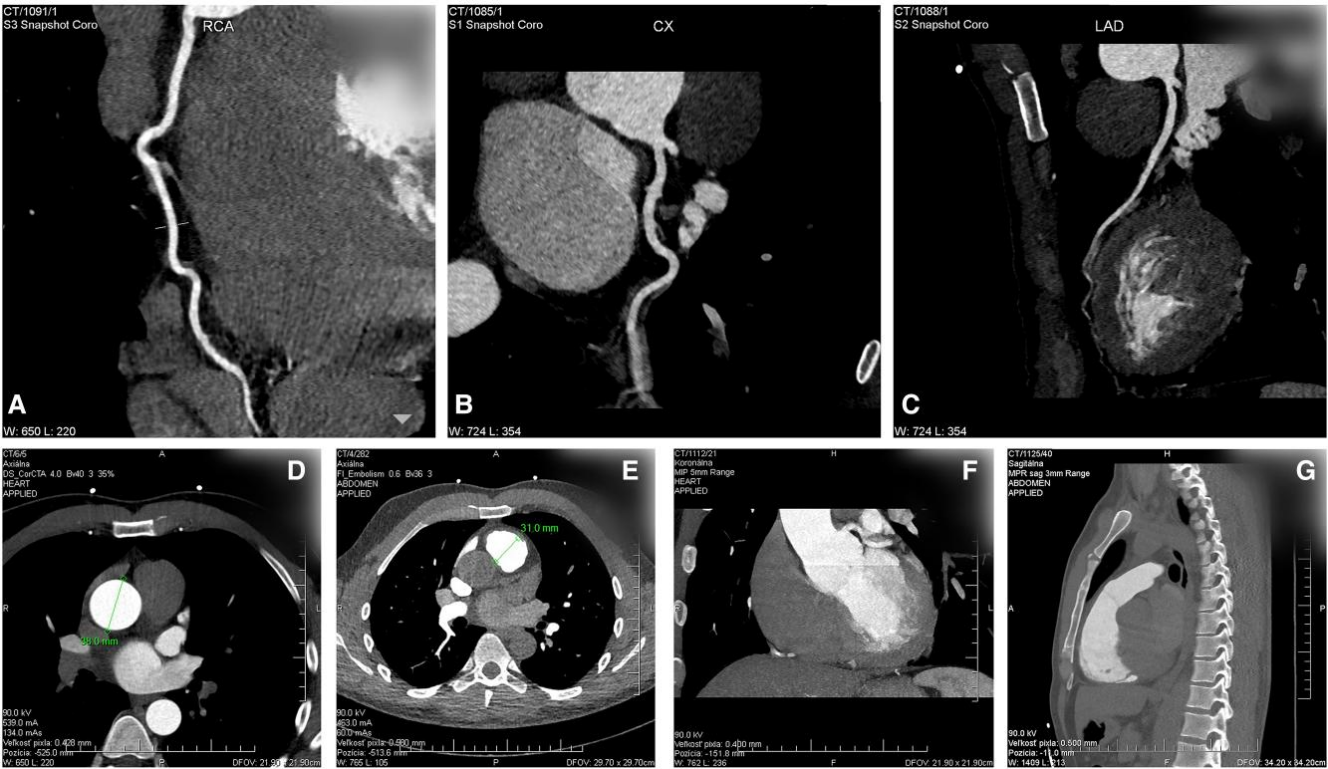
Computed tomography images focused on the anatomy of the coronary tree and the diameters of the aorta and pulmonary artery: (*A*) right coronary artery; (*B*) circumflex artery; (*C*) left anterior descending artery; (*D*) aorta diameter; (*E*) pulmonary artery diameter; (*F*) anatomy of the aorta; (*G*) anatomy of the pulmonary artery.
